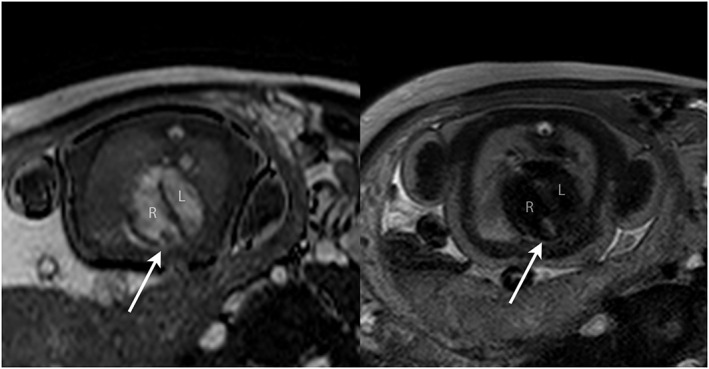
Balanced SSFP bright blood image (A) and single‐shot fast spin‐echo (SSFSE) black‐blood image (B) in a 38‐week fetus with an RV mass (arrowed). Based on the size, position and tissue signal on multiple sequences, a solitary ventricular rhabdomyoma was suspected; no other masses were seen including on concomitant fetal brain MRI. All findings were confirmed postnatally. R, right ventricle; L, left ventricle
